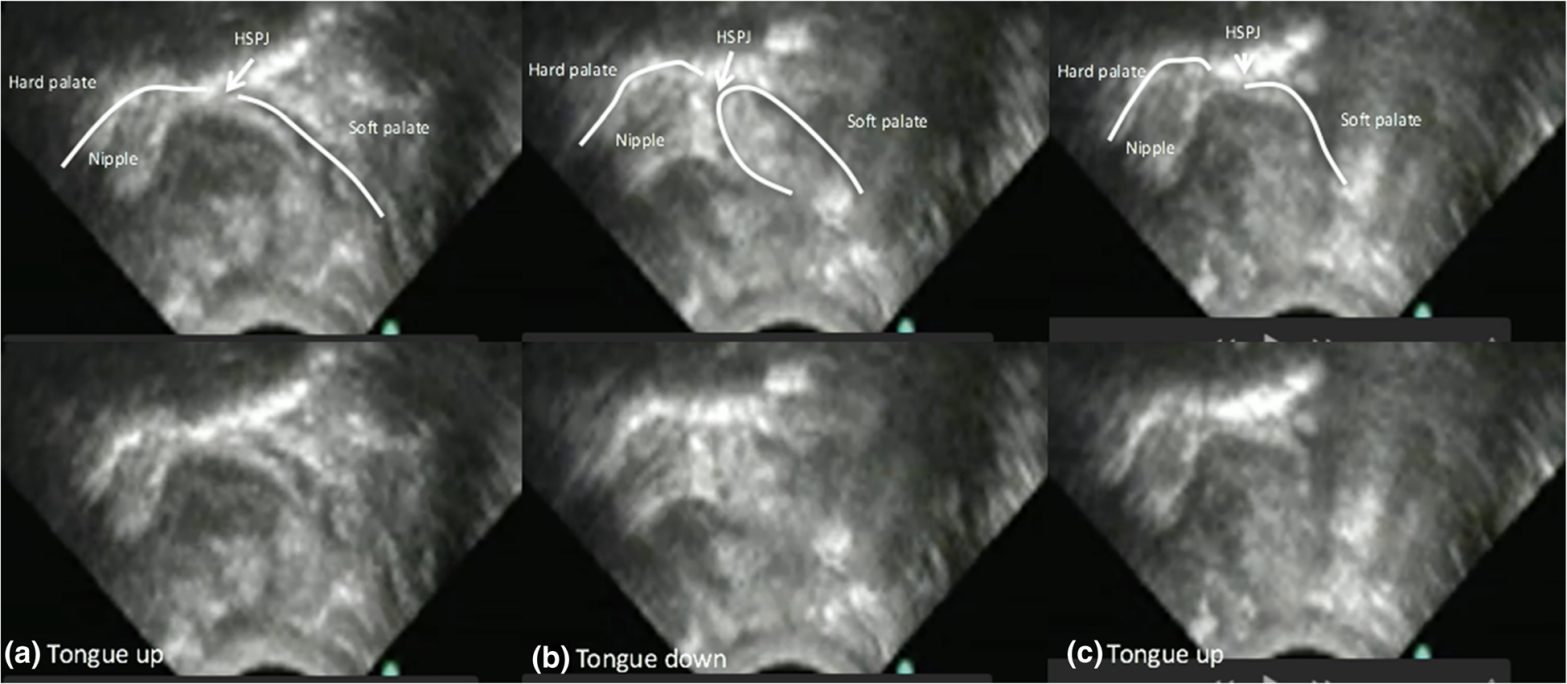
Ultrasound images of one cycle of a preterm breastfeeding infant (a) tongue up corresponds with baseline vacuum (b) when the tongue is lowered to the lowest point peak vacuum is applied to the breast and milk flows (c) tongue returns to the soft palate and milk is removed from the oral cavity
